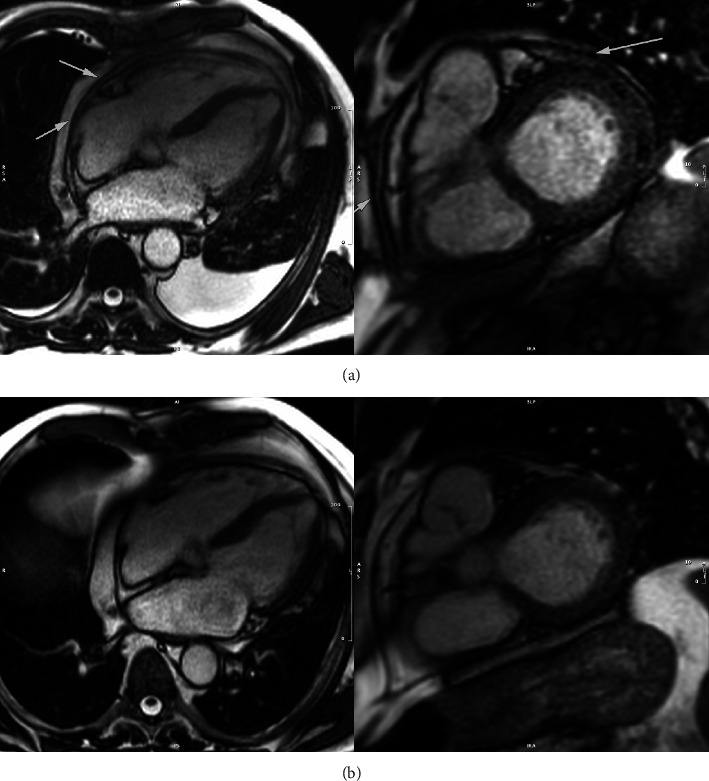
Cardiac MRI images (case 1). (a) Images of postpericardiocentesis showing pericardial thickening with pleural effusion (arrows). (b) Eight weeks later following treatment with steroids and colchicine.
